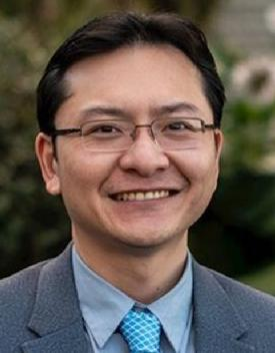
Dr Shaw Hua (Anthony) Kueh is a Cardiologist at Auckland City Hospital, New Zealand. He graduated from the Dunedin School of Medicine at the University of Otago and completed his Cardiology training at Green Lane Cardiovascular Services. He was supported by the New Zealand Heart Foundation to undergo fellowship training in cardiac CT and MRI at St Paul’s Hospital, Vancouver, Canada. He has a particular interest in structural imaging and is a mentor and supervisor for trainees of the Royal College of Physicians.
